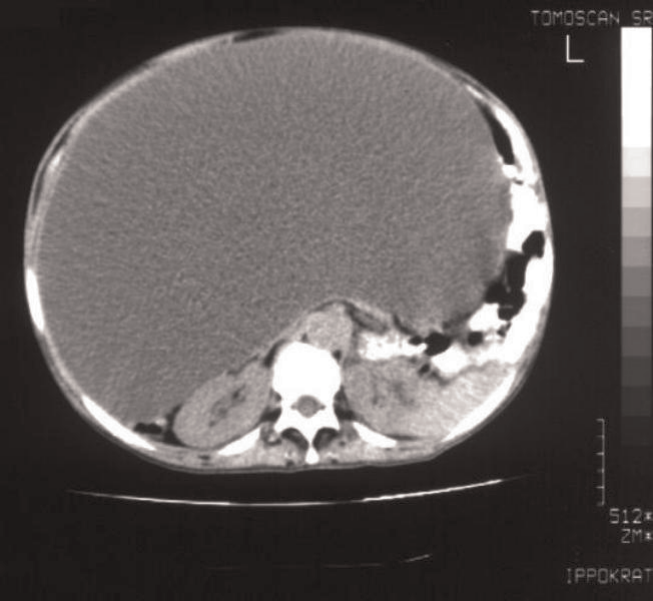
Initial computed tomogram of the abdomen of the patient with a giant ovarian cyst mimicking ascites.
